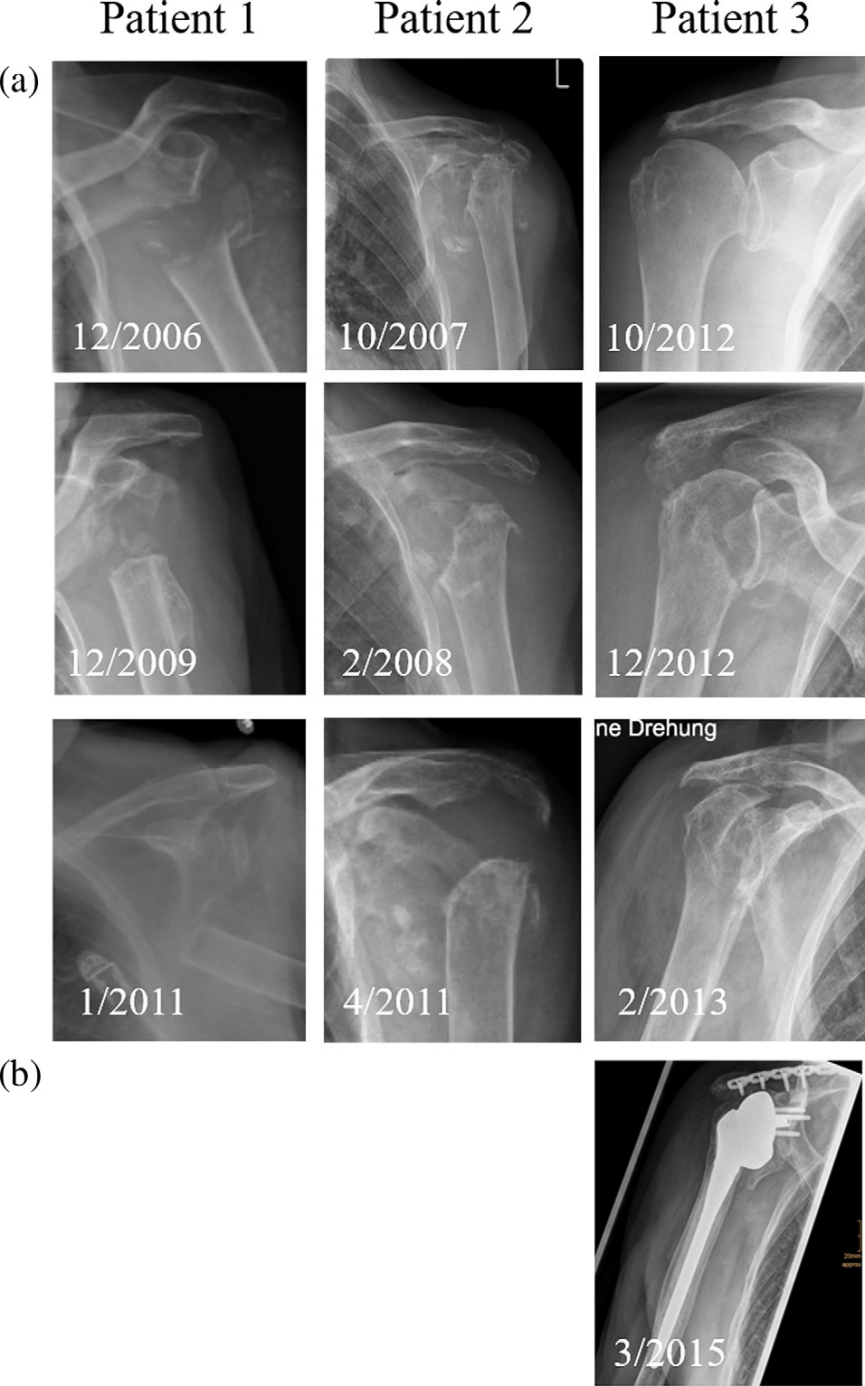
Antero-posterior radiographs of the involved shoulder of each of the three patients over time: (a) progressive osteolysis in all three patients; (b) implanted reverse shoulder prosthesis without loosening 12 months postoperatively.
